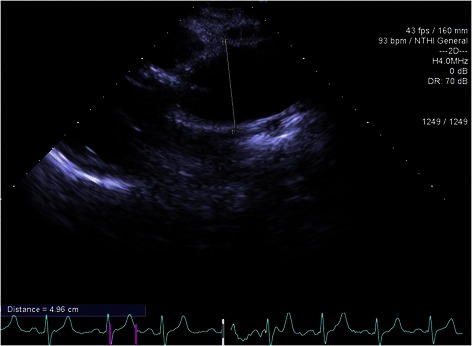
Case report 2, transthoracic echocardiogram parasternal long axis- ascending aorta before the operation
